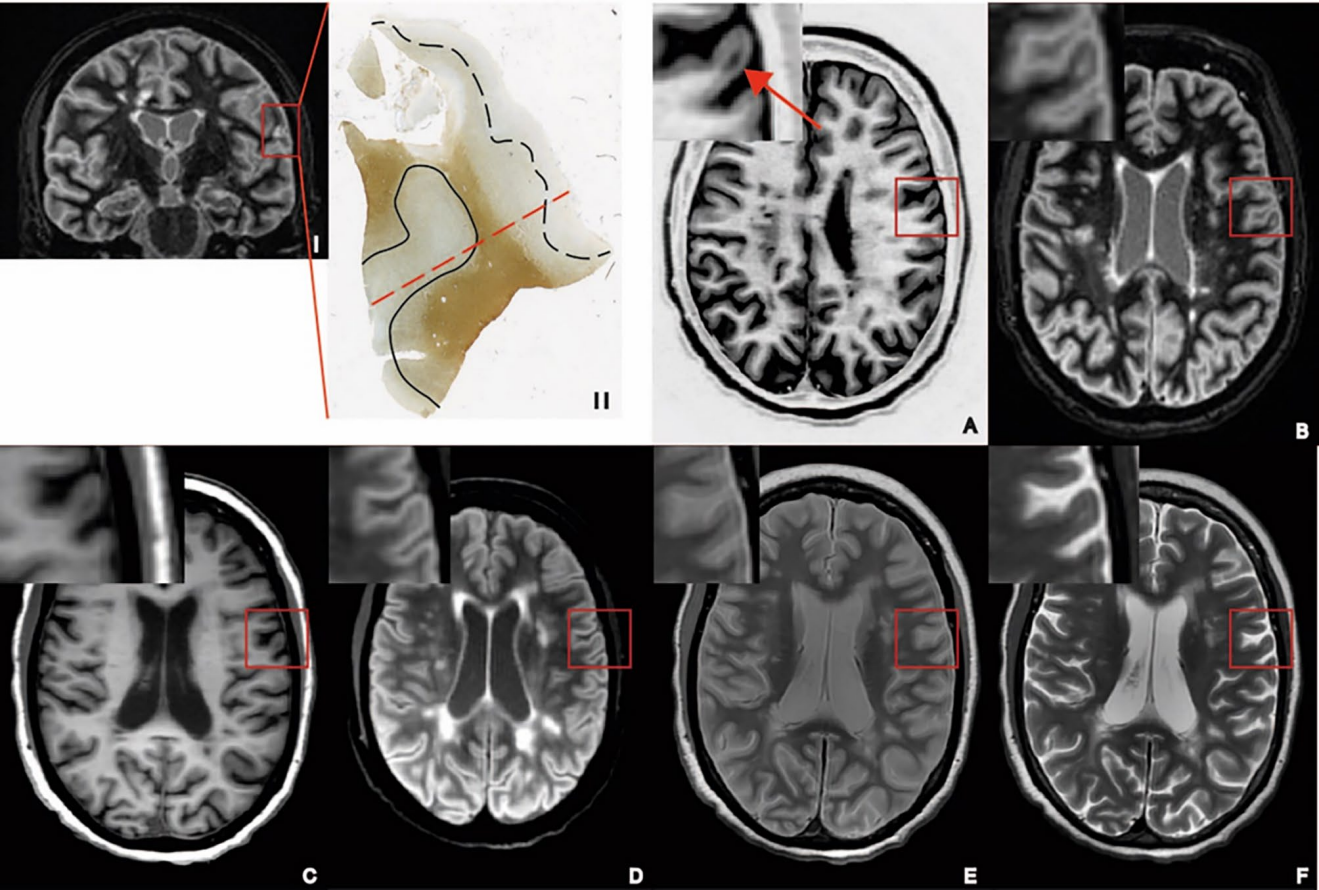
Example of a type IV lesion that is discernible on DIR and PSIR but less to not on other sequences. (I) Coronal double inversion recovery with inset of tissue sample. (II) Tissue sample in which a type IV lesion is demarcated by the black line. The dotted black demarcates a type III lesion that is not discernible on any of the sequences. The red line indicates the level of the axial MRI images in the tissue sample. A Phase-sensitive inversion recovery image in which the lesion is discernible. B Double inversion recovery image in which the lesion is discernible. C 3D-T_1_ weighted. D Fluid-attenuated inversion recovery. E Proton density. F T_2_ weighted
